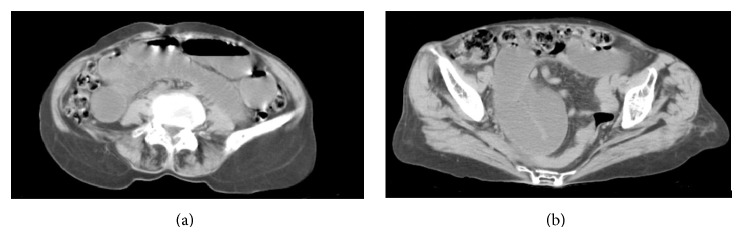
Plain CT scan of lower abdomen and pelvis showing dilated small bowel loops in abdomen and pelvis.
